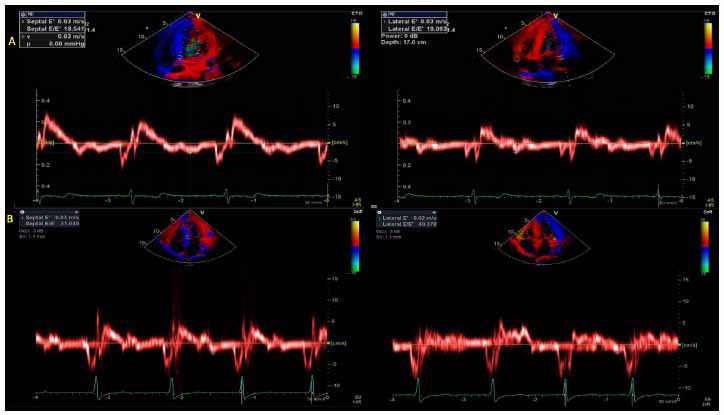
Tissue Doppler velocities measured at the septal and lateral annulus from the same young non-HOCM variant patient (row A) and elderly HOCM patient (row B), both with increased E/e’ ratio (>14), consistent with elevated mean LA pressure.
